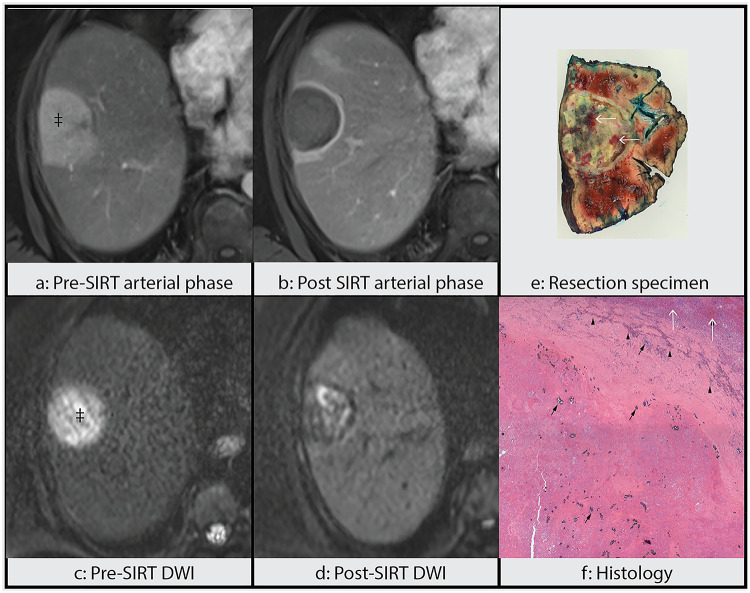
a–f Tumour (‡) demonstrating loss of arterial enhancement (a, b) but residual abnormal signal on DWI (5c, 4 d) corresponding to regions of intratumoral hemorrhage (white arrows 4e and 4f) in otherwise complete necrotic tumour with SIRT beads (short arrows) and hemosiderin depositation (arrowheads) (f)
